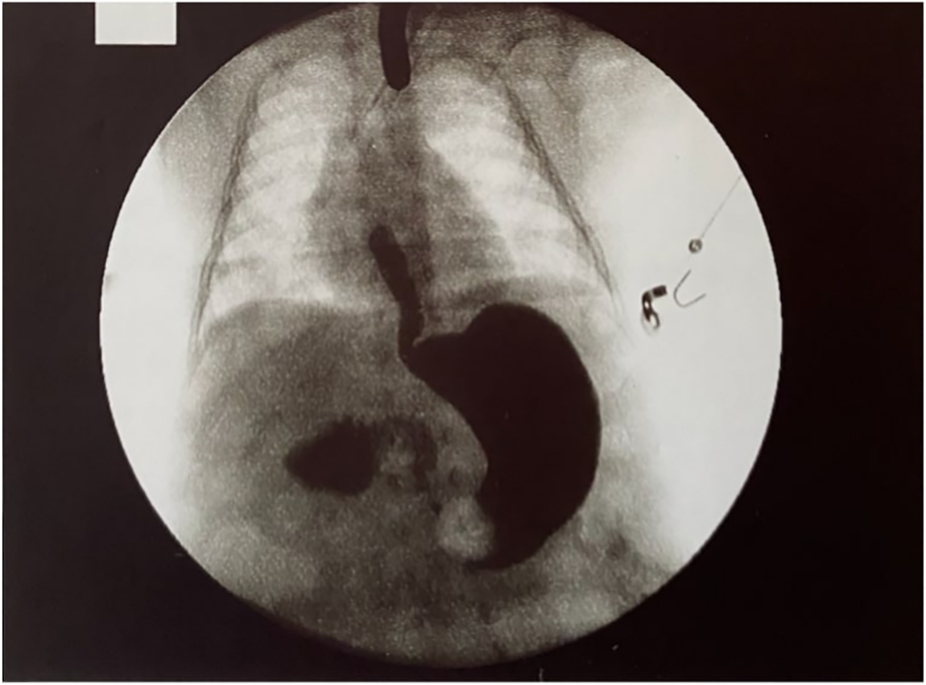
X-Ray evaluation of the distance between upper and lower pouch.
